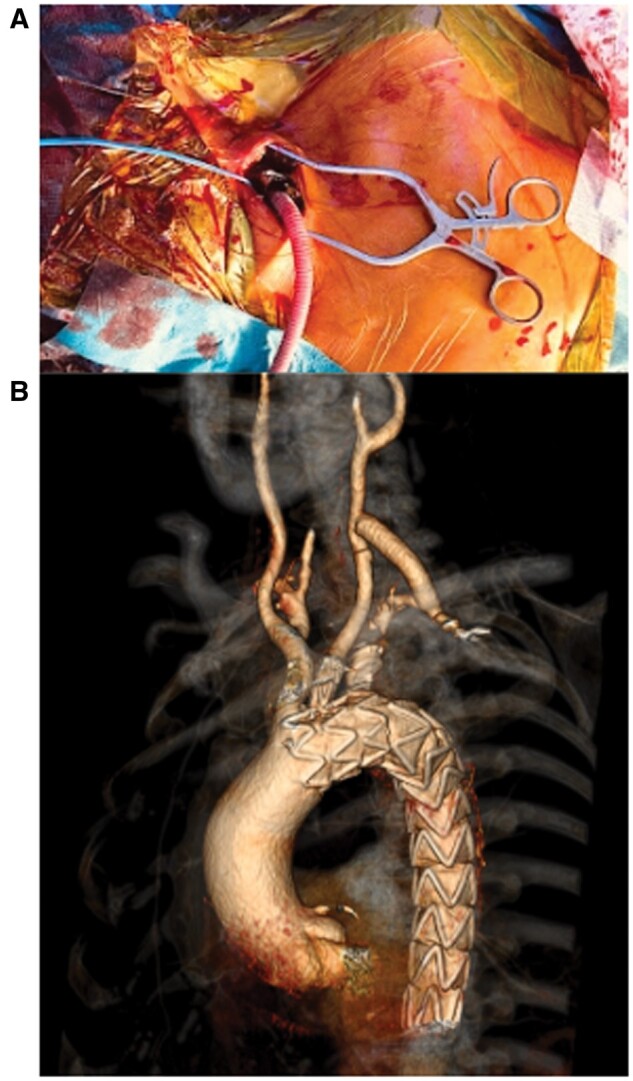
(A) Vascular access was created through an 8-mm Dacron graft directly anastomosed to the LCA. The Dacron graft was used at the end of the procedure to create a left carotid-subclavian bypass. (B) Computed tomography angiography scan taken at 3 months.
